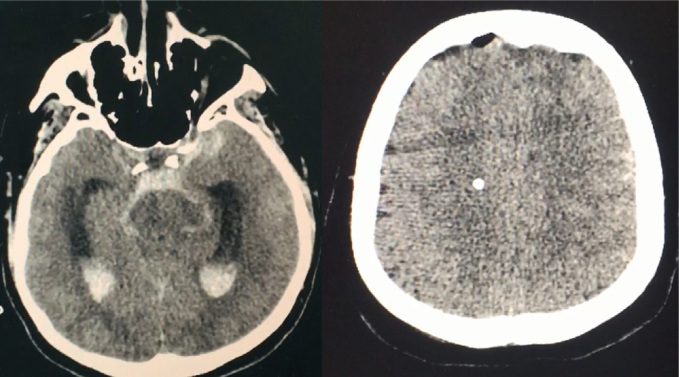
Computed tomography findings in intracranial hypertension (A) loss of cortical-subcortical differentiation and cortical subarachnoid hemorrhage. (B) temporal horn dilation and cisternal subarachnoid hemorrhage.
